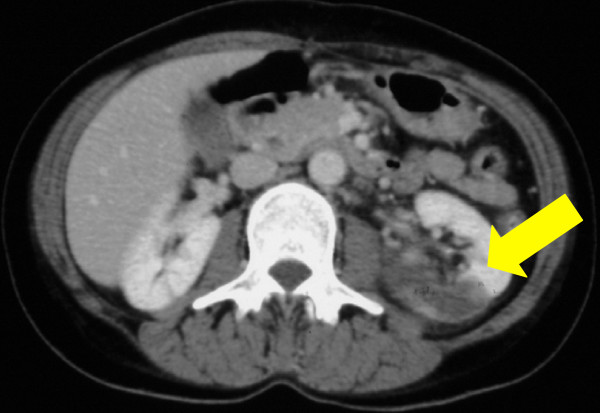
Contrast-enhanced abdominal computed tomography. Yellow arrow indicates left renal infarction.
